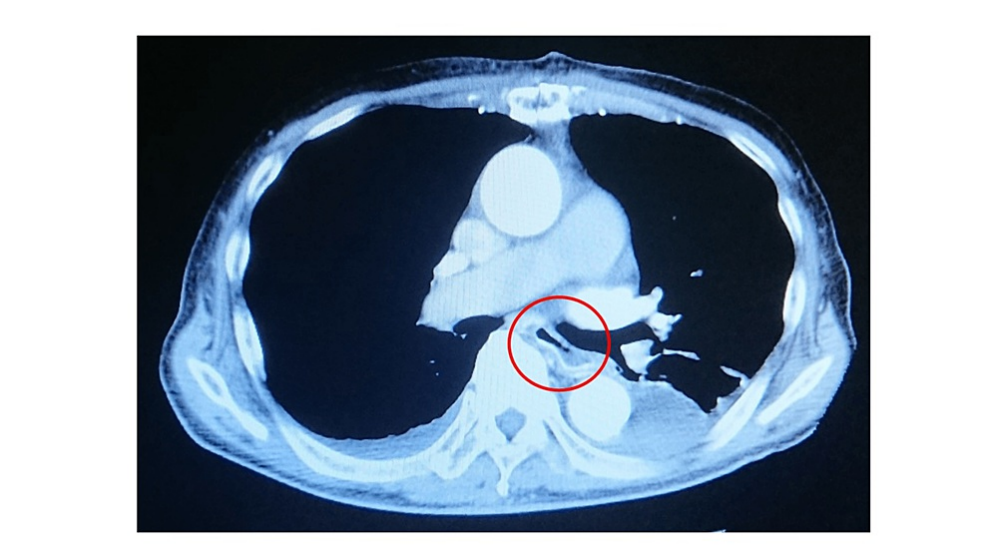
Chest CT image, axial view, taken on postoperative day 11. The giant submucosal esophageal hematoma has resolved. CT: computed tomography
